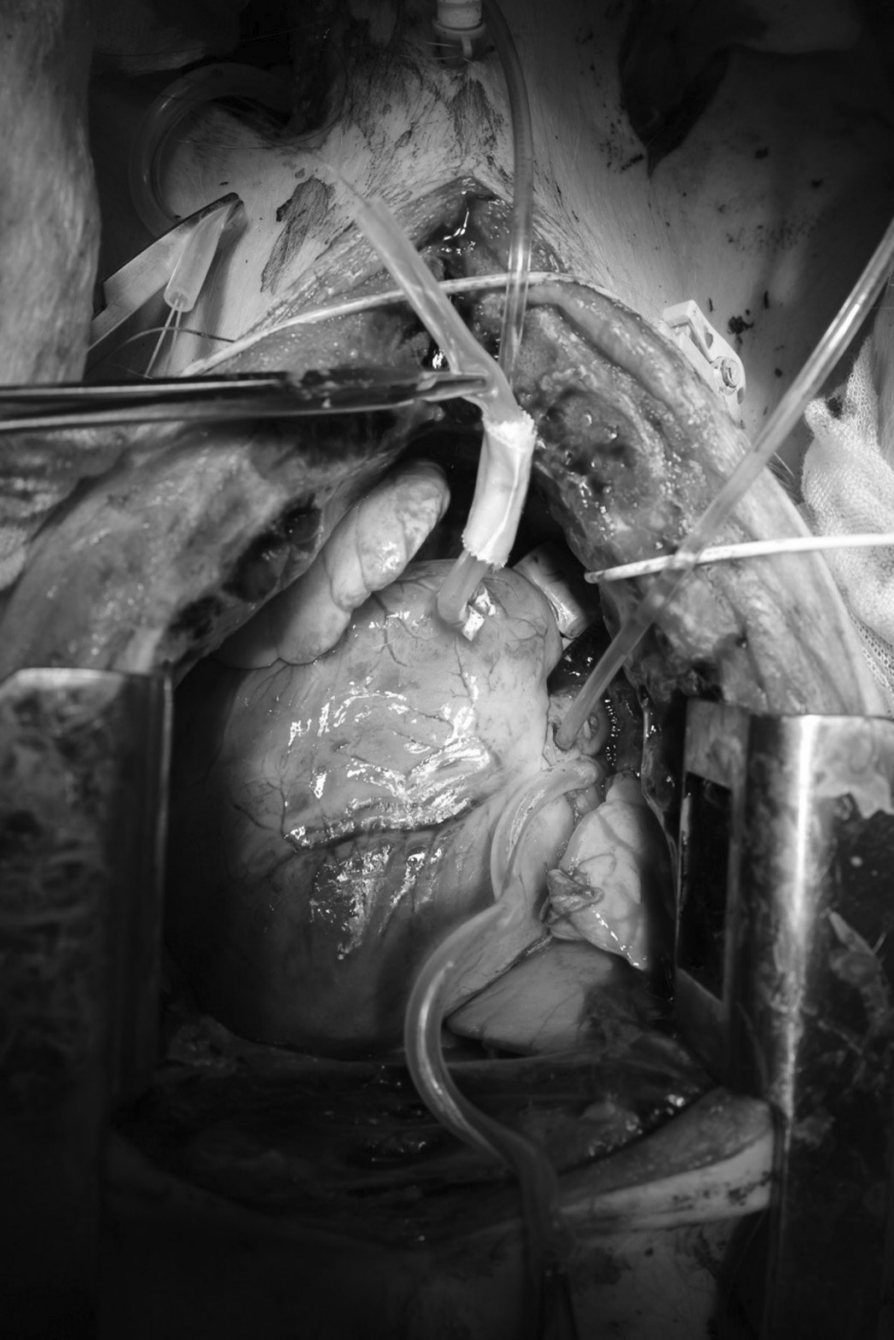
The ultrasonic probe around the pulmonary artery and the pressure probes in the left atrium and pulmonary artery.
